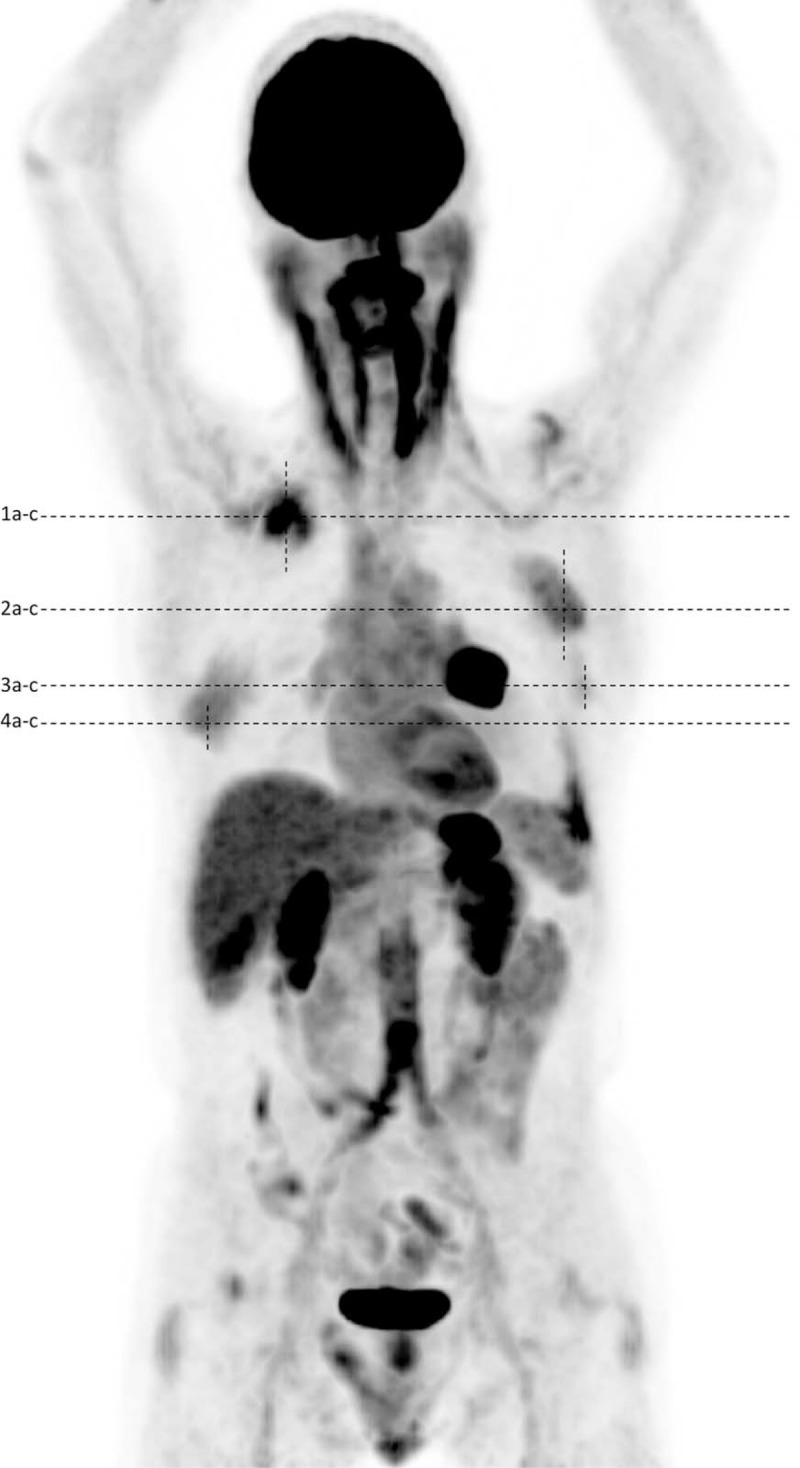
MIP image of the Fluorodeoxyglucose-positron emission tomography-computed tomography (^18^F-FDG-PET) acquisition. The highest SUV_max_ measured on elastofibromas dorsi was 4.7 (Fig. 1 1images 1a-c). This image also shows an intense uptake of an aortoiliac stent 16 years after surgery, probably because of an infection.
